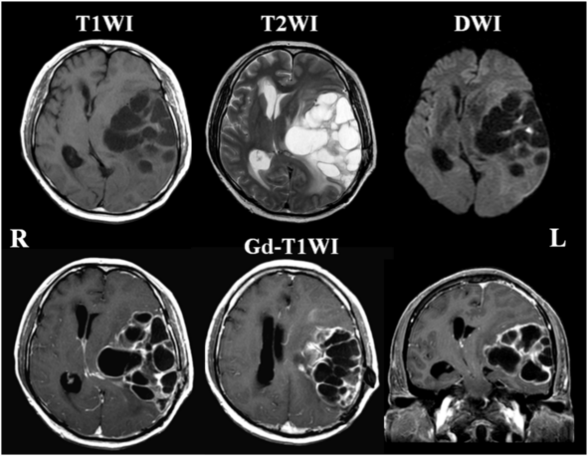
MRI 3 months after treatment The size and number of the cystic lesions have increased with a pronounced left to right midline shift demonstrating impending cerebral herniation. Enhanced lesions still localized surround the cysts and there is no evidence of tumor extension into the basal ganglia or corpus callosum
